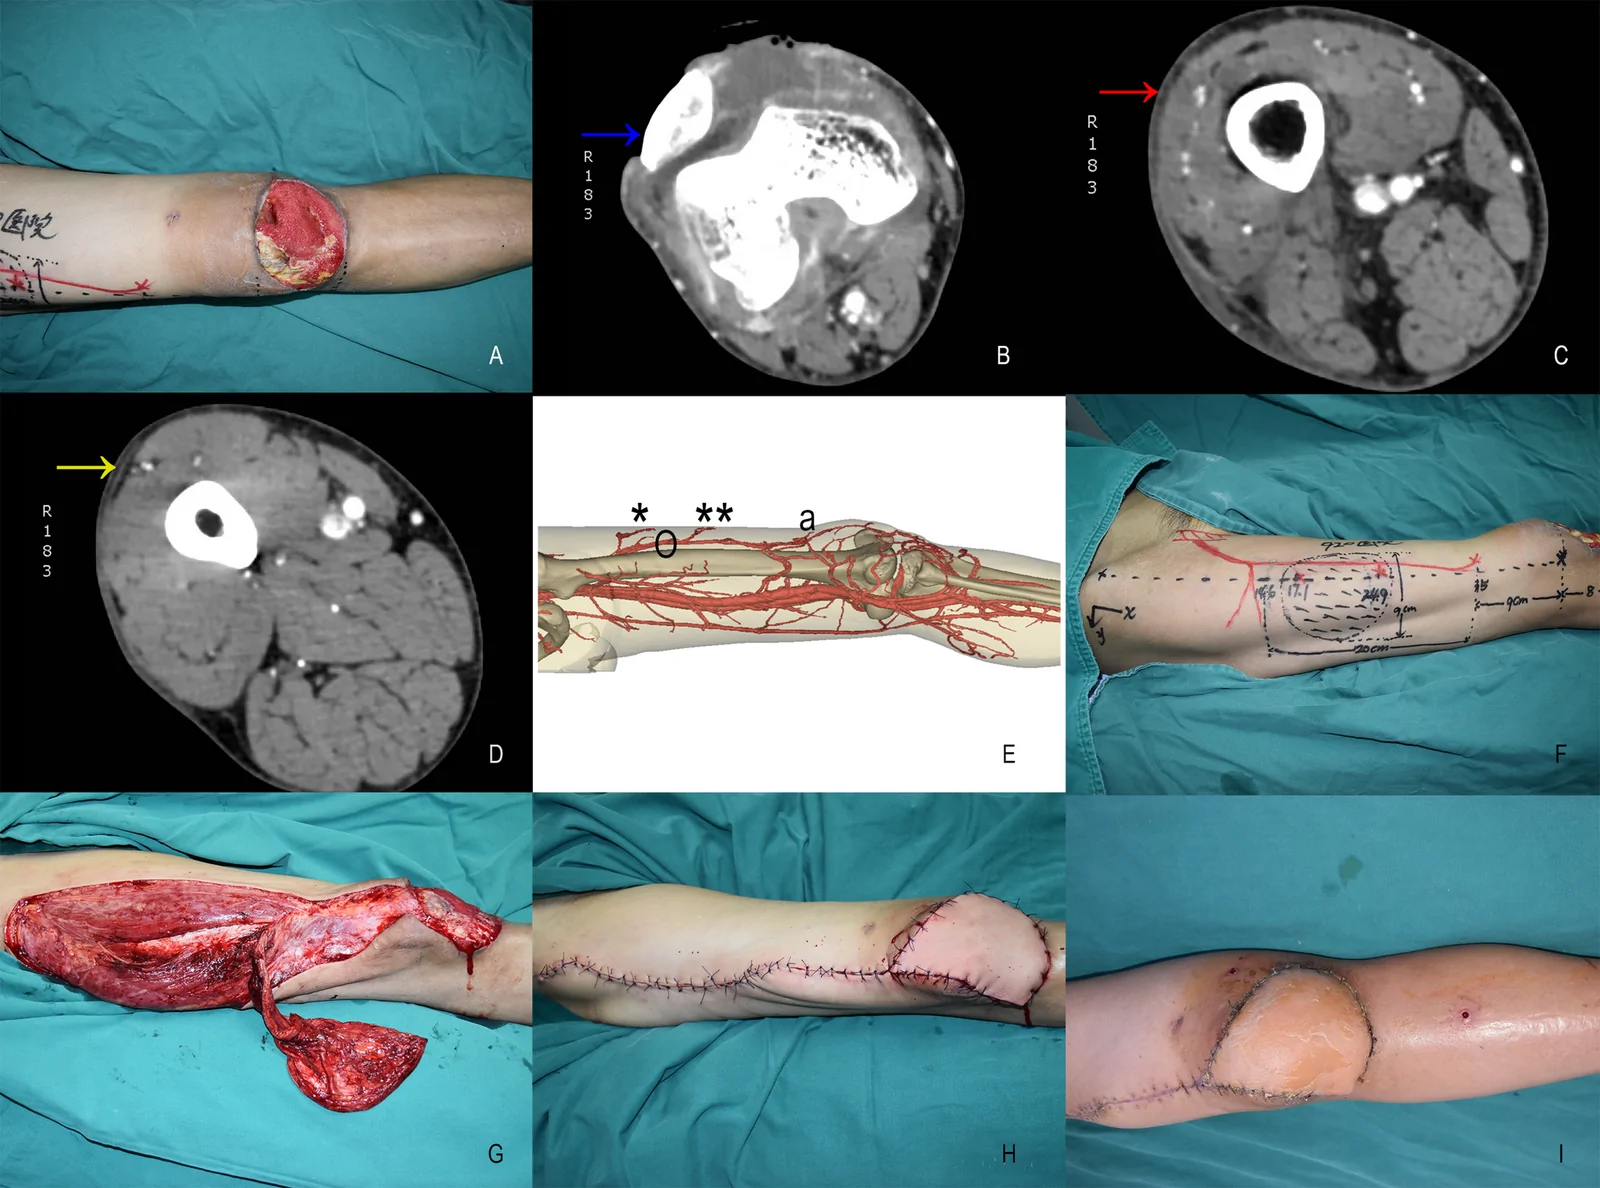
(A) Preoperative view showing a transverse soft tissue defect over the anterior aspect of the right knee. B Preoperative CTA image showing the location of the soft tissue defect, indicated by the blue arrow. The defect measured 9 × 8 cm, with patellar exposure. C Preoperative CTA image showing an anastomotic connection between the descending branch of the lateral circumflex femoral artery and the periarticular arterial network around the knee, indicated by the yellow arrow. D Preoperative CTA image showing the location of perforator 2, indicated by the red arrow. E Mimics 20.0 reconstruction demonstrating two perforators arising from the descending branch of the right lateral circumflex femoral artery. O, source vessel; *, perforator 1; , perforator 2; a, anastomotic branch. F Design of a retrograde anterolateral thigh flap based on perforator 2. G Intraoperative view after harvest of a 14 × 9 cm retrograde anterolateral thigh flap without muscle dissection. H Immediate postoperative appearance after flap inset. I One-month postoperative photograph showing early satisfactory flap contour and texture
